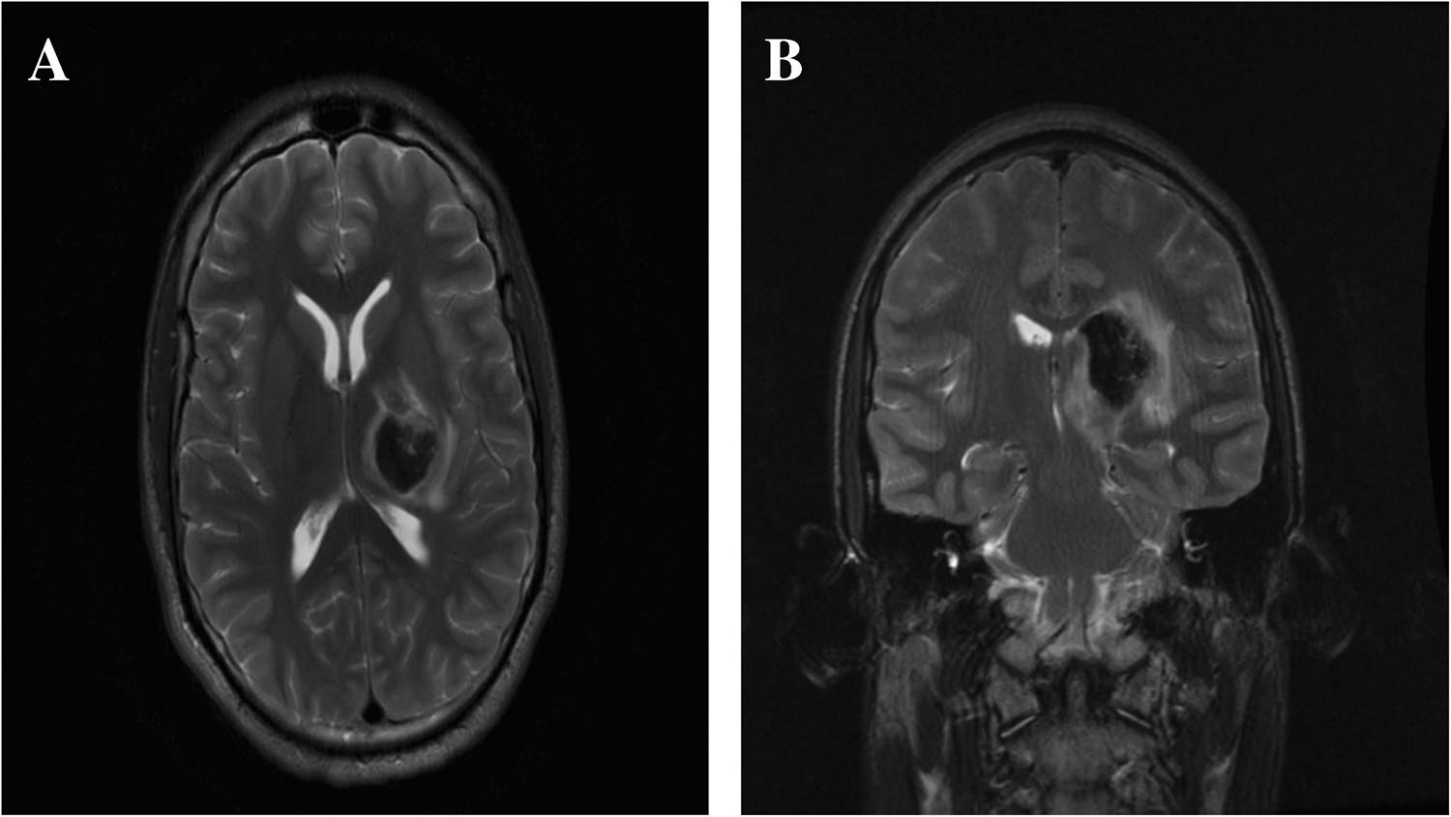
Magnetic resonance imaging (A axial; B coronal) demonstrating a large left thalamic haemorrhage
